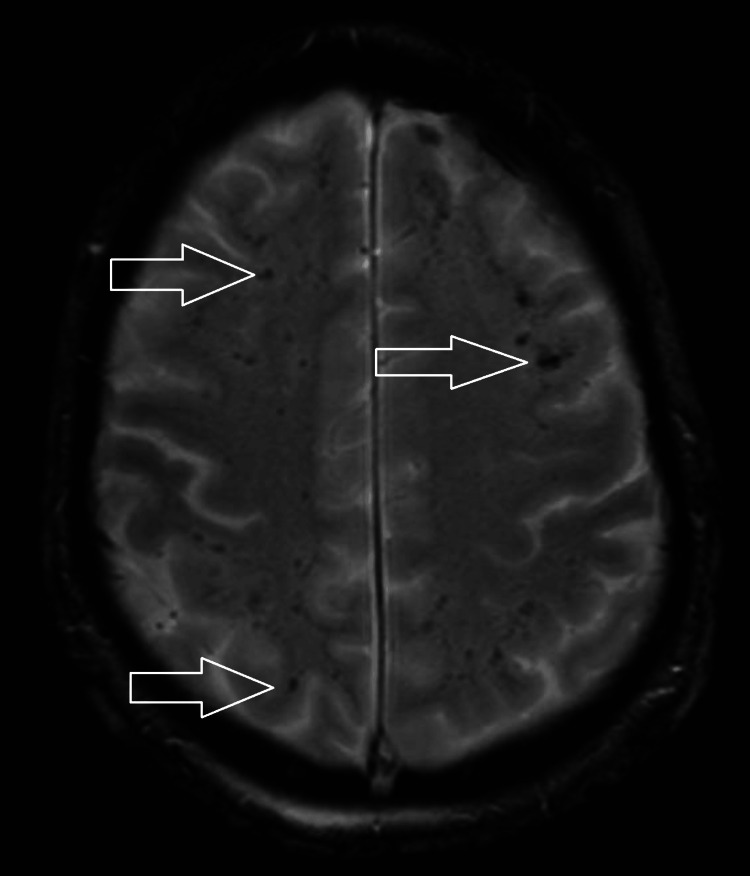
MRI head axial view. Image from the susceptibility-weighted sequence showing innumerable foci of increased susceptibility indicating microhaemorrhages.
